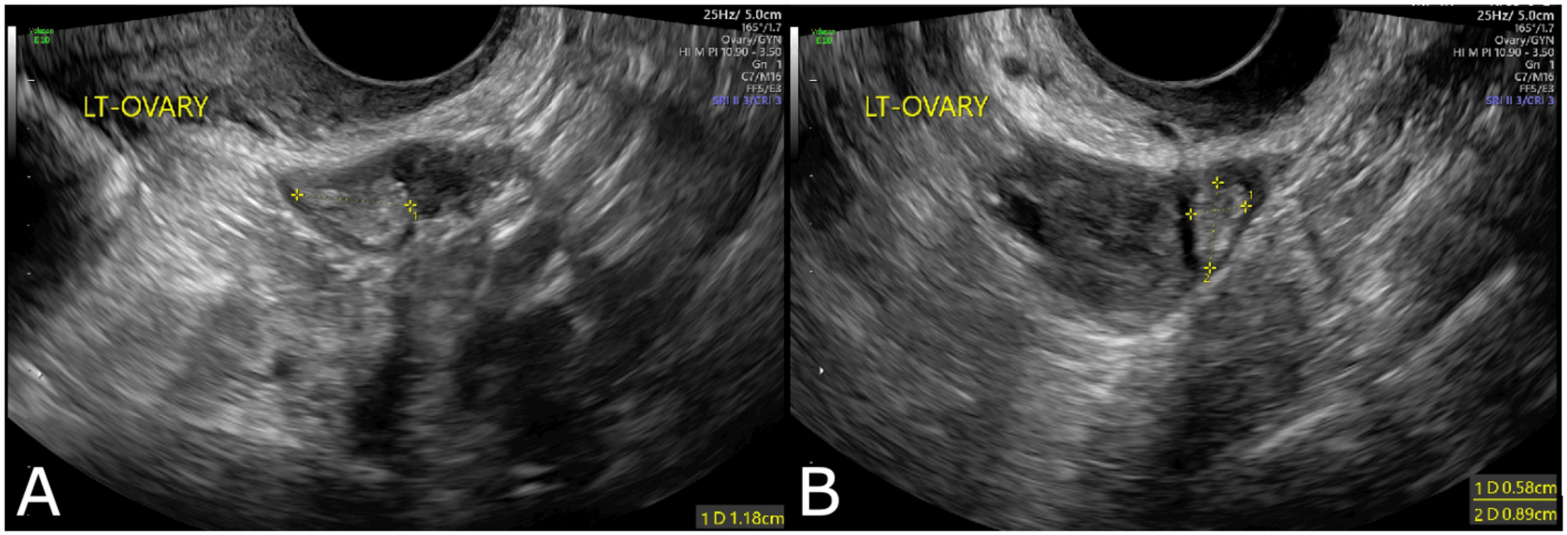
Repeat Pelvic Ultrasound Repeat pelvic ultrasound demonstrating hemorrhagic structure adjacent to the left ovary measuring 1.18 x 0.58 x 0.89 cm.
